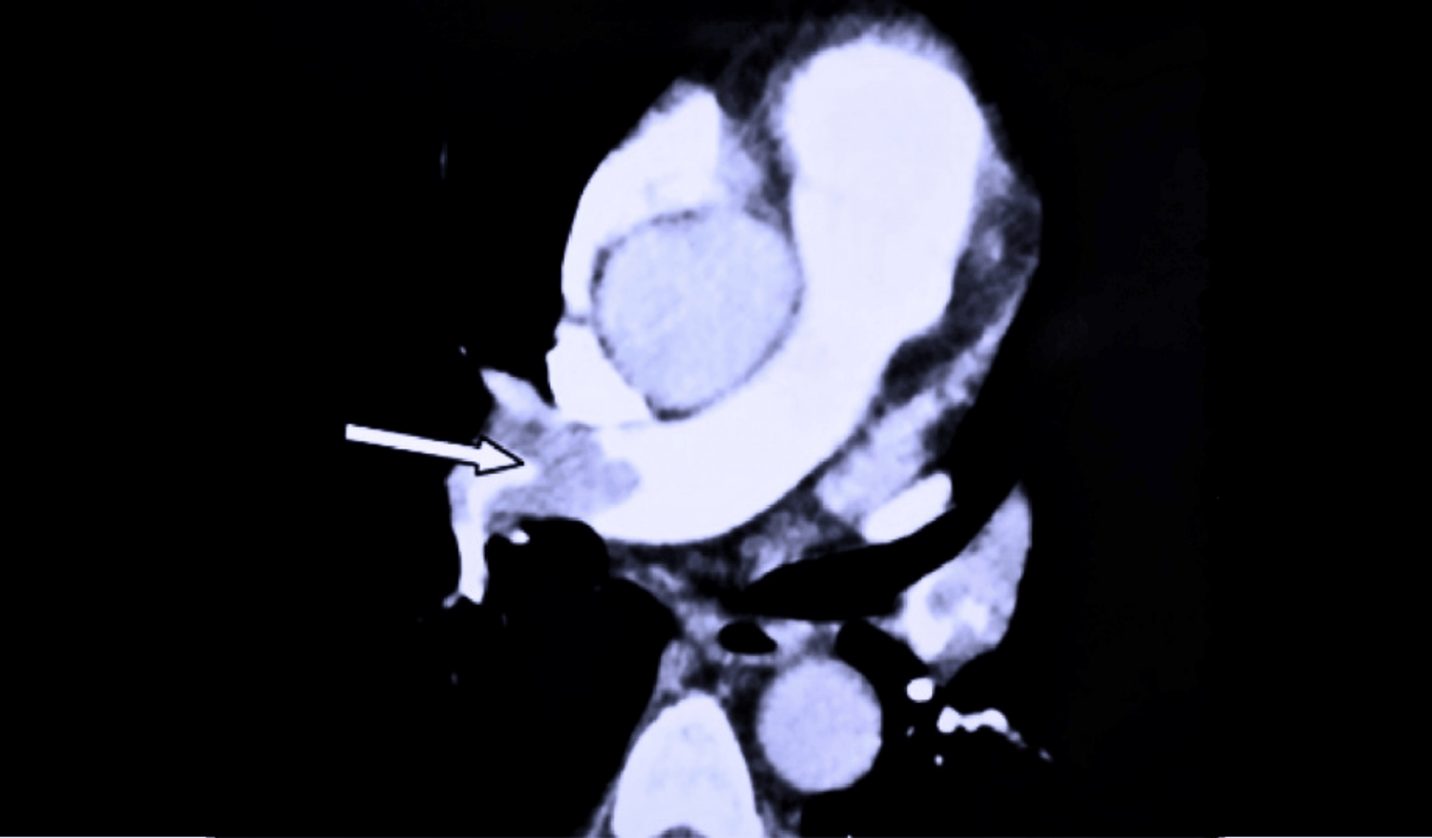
CT pulmonary angiogram: extensive pulmonary artery embolism involving the right pulmonary artery and segmental branches of the right lower lobe
